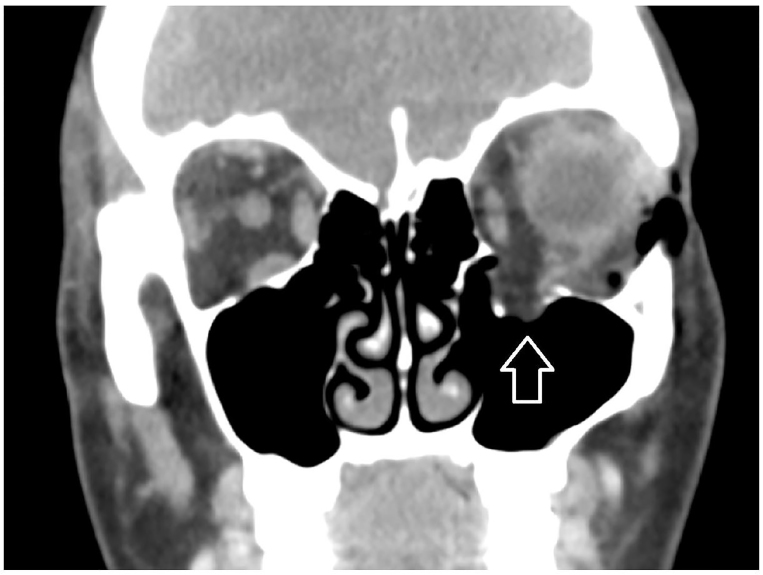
Computed tomography shows left orbital floor blowout fracture with herniation of the orbital fat, inferior rectus muscle and inferior oblique muscle into the left maxillary sinus (arrow).
